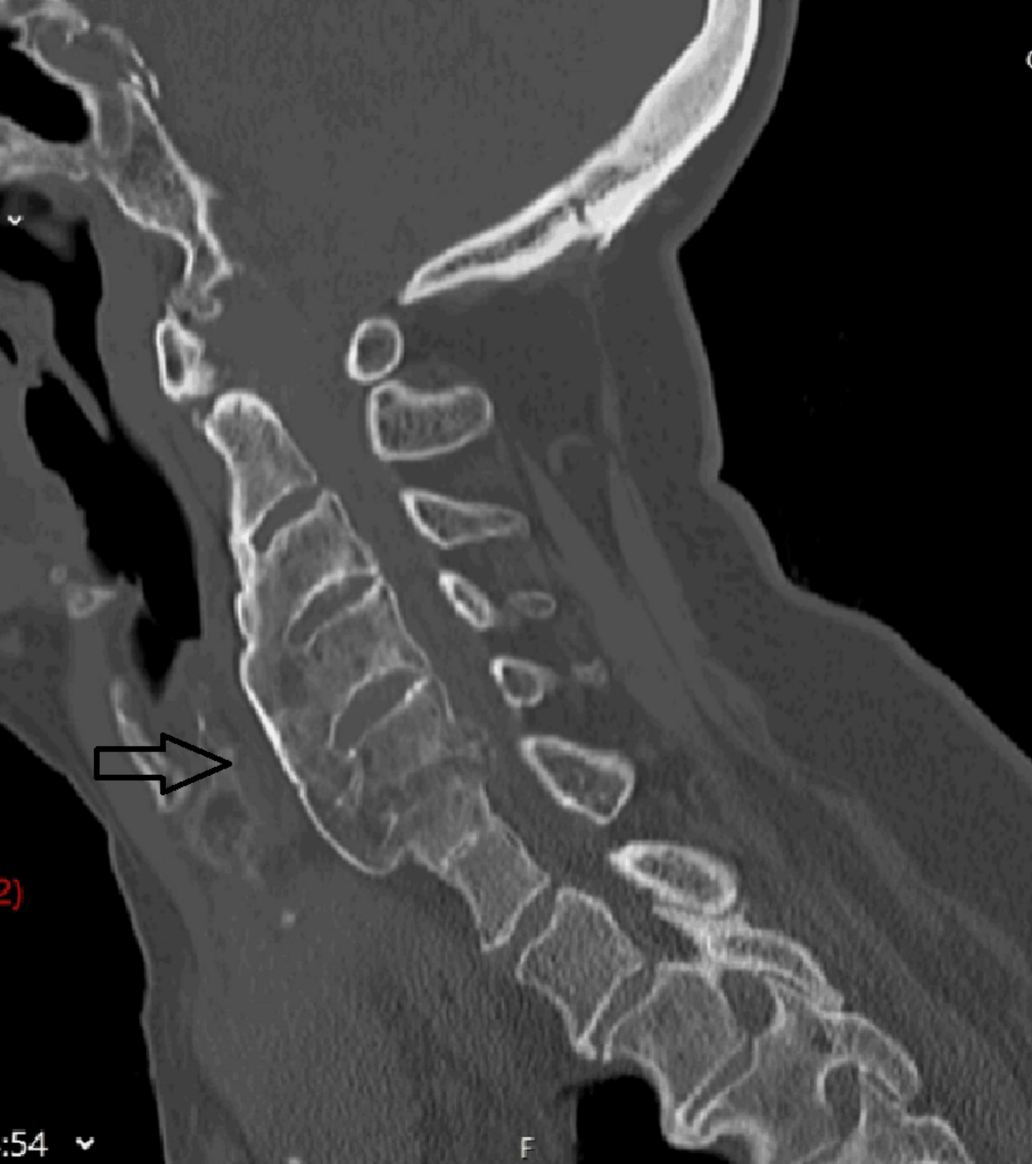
CT scan before surgery. Arrow shows C3-C6 diffuse idiopathic skeletal hyperostosis
